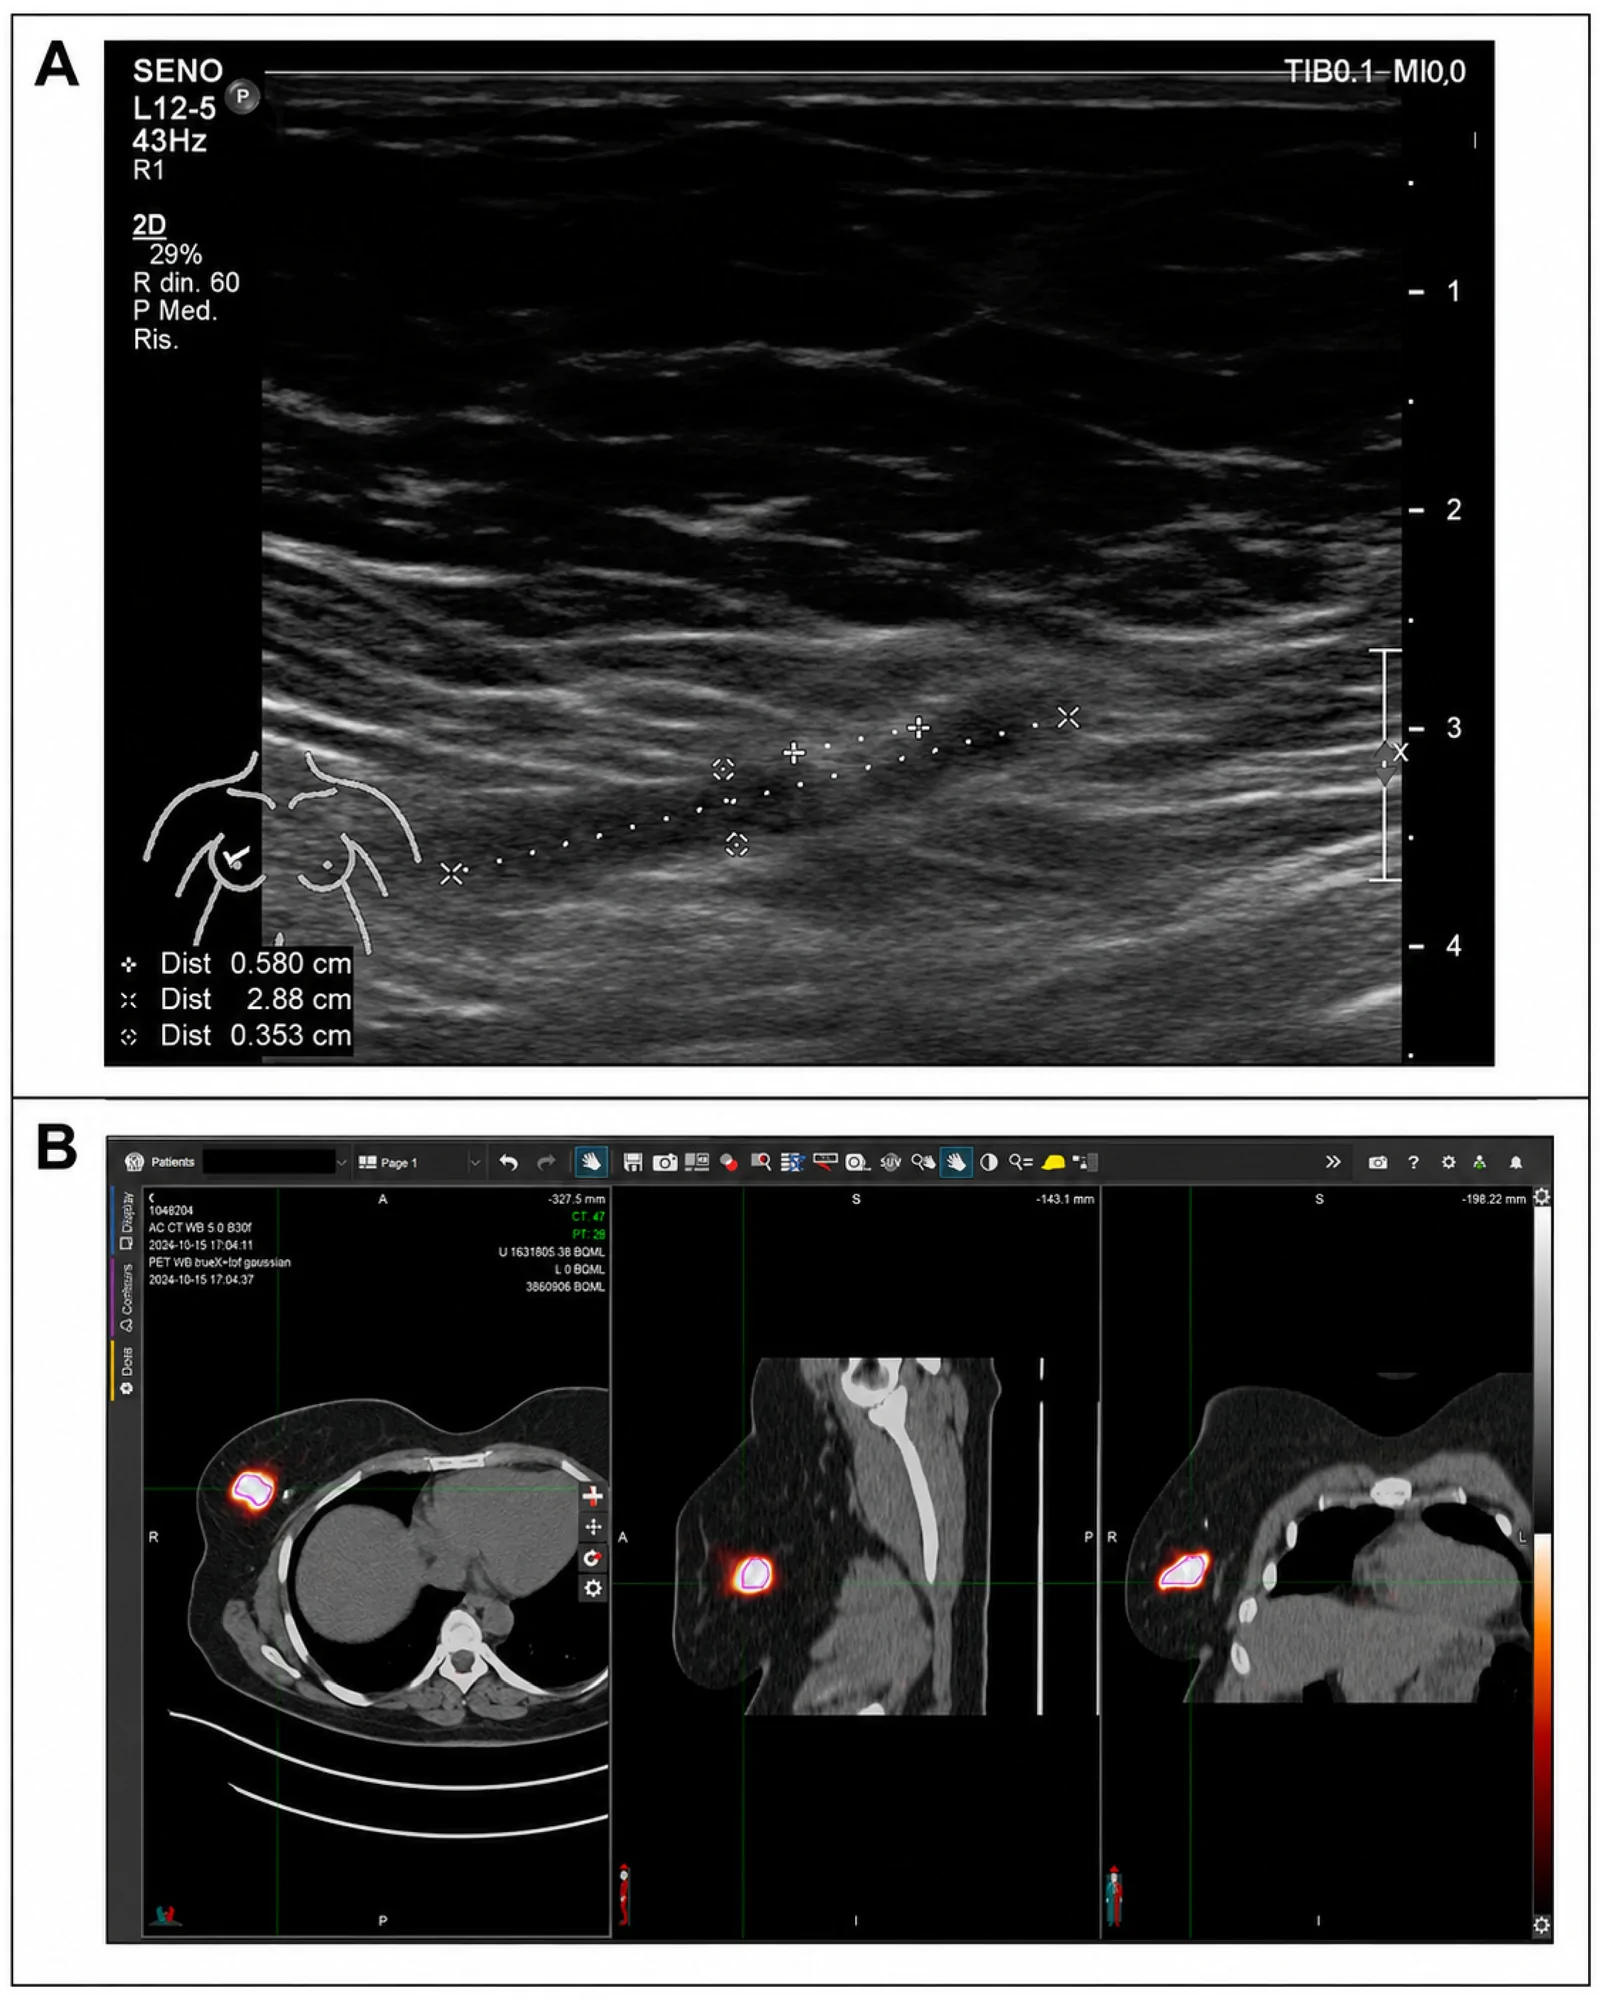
Ultrasound-guided treatment administration and early focal localization on ^90Y PET/CT. (A) Ultrasound image showing the post-VABB target region during ultrasound-guided intralesional treatment. (B) Dedicated breast ^90Y PET/CT acquired approximately 1 h after administration, demonstrating early focal activity centered within the treated post-VABB target region, without clinically relevant off-target uptake on qualitative assessment. In the PET/CT panel, the color scale represents relative ^90Y activity intensity, with warmer colors indicating higher activity; crosshairs indicate the focal activity localization. Ultrasound calipers and symbols represent standard imaging-system measurement annotations.
